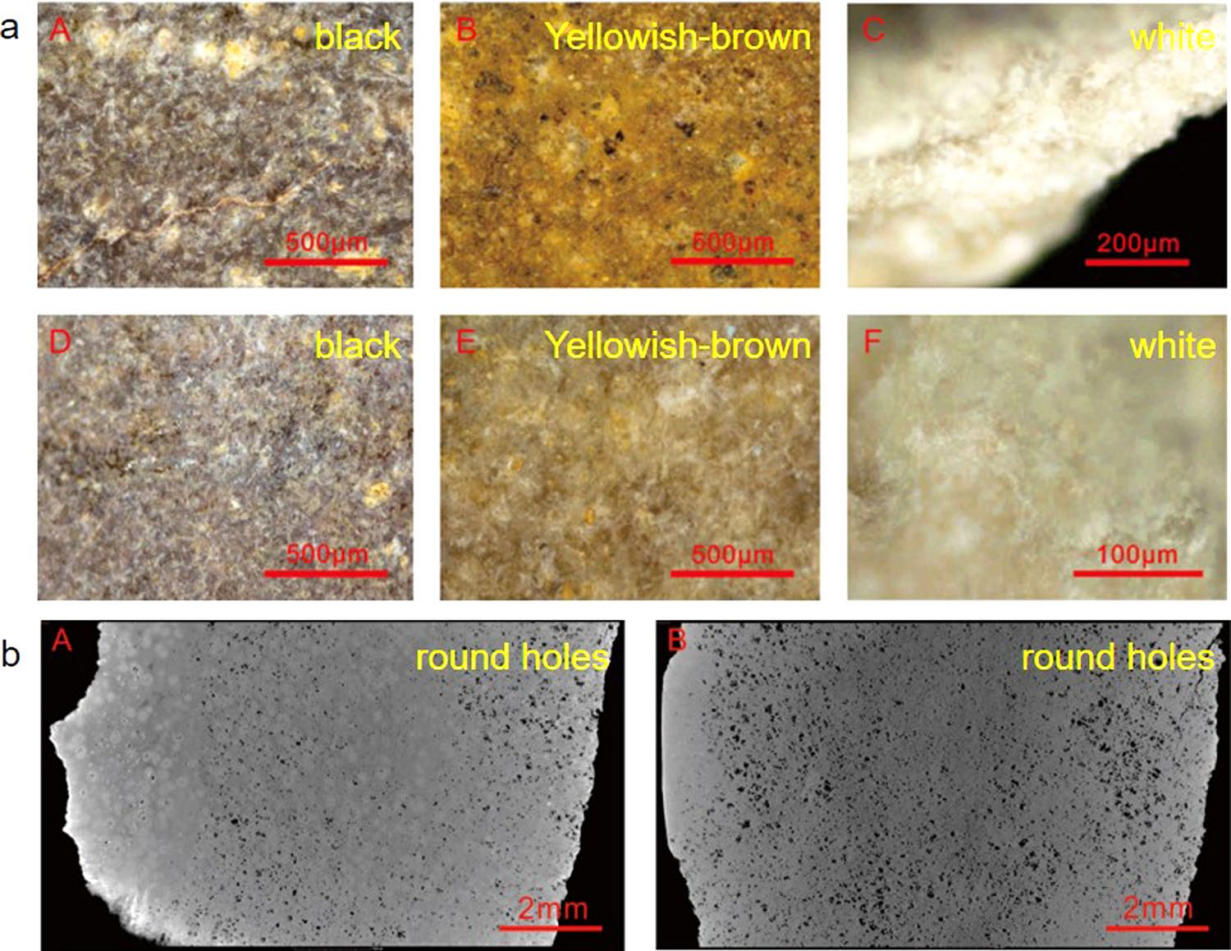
The micro photos and CT images of G212-1 and G21:2-2. (a) The micro photos. (A,B,C) the micro photos of G212-1. (D,E,F) the micro photos of G212-2. (b) The CT images of G212-1.
